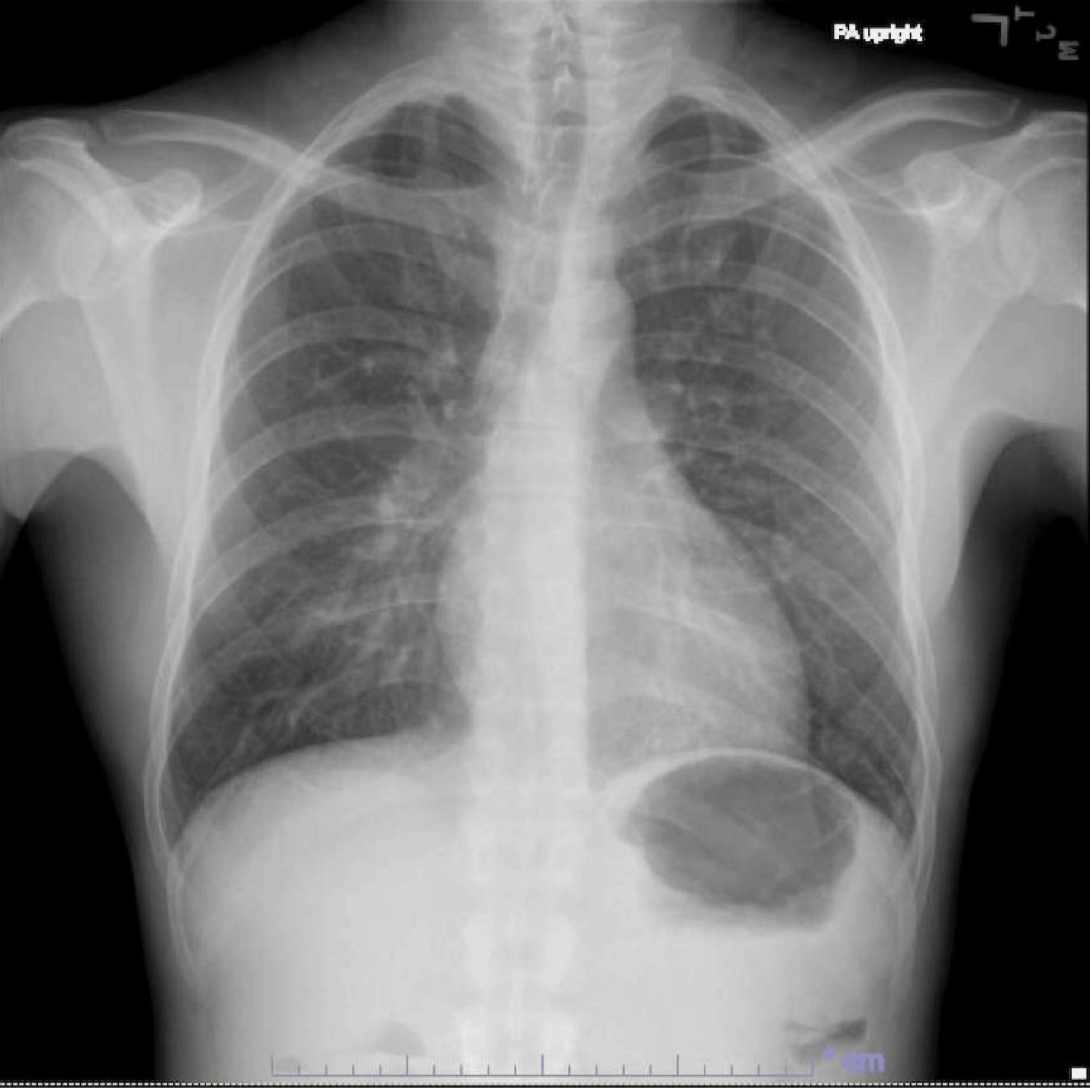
Chest X-ray demonstrating mild bronchial wall thickening without significant consolidation. The chest X-ray shows mild bronchial wall thickening with no evidence of consolidation, pleural effusion, or pneumothorax. The findings are suggestive of an early or mild infectious process, consistent with the patient’s clinical presentation of chronic cough and immunosuppression in the context of untreated HIV.
